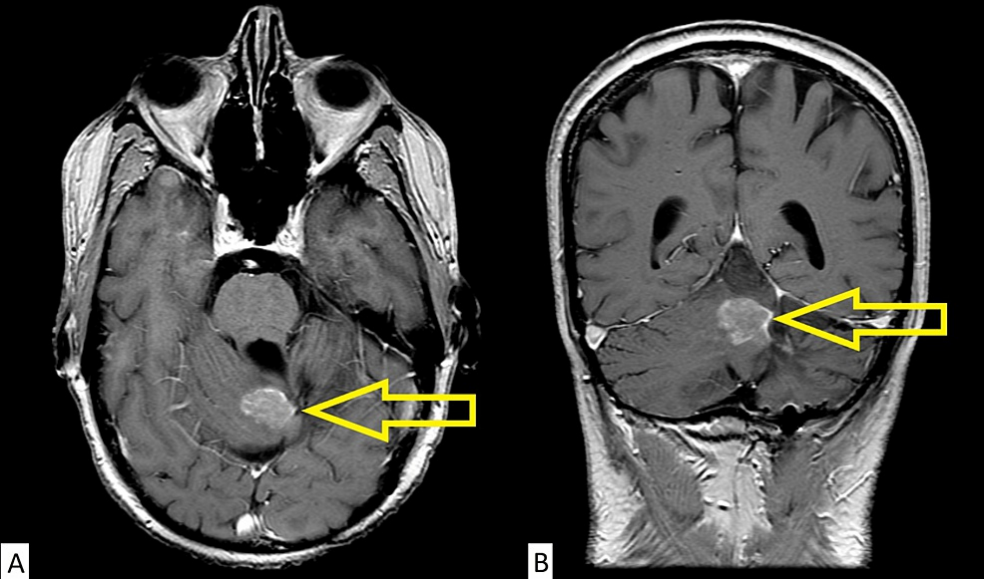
Restaging MRI. Gadolinium-enhanced T1-weighted (A) axial and (B) coronal images of the brain demonstrating local recurrence within the vermis. MRI, magnetic resonance imaging
